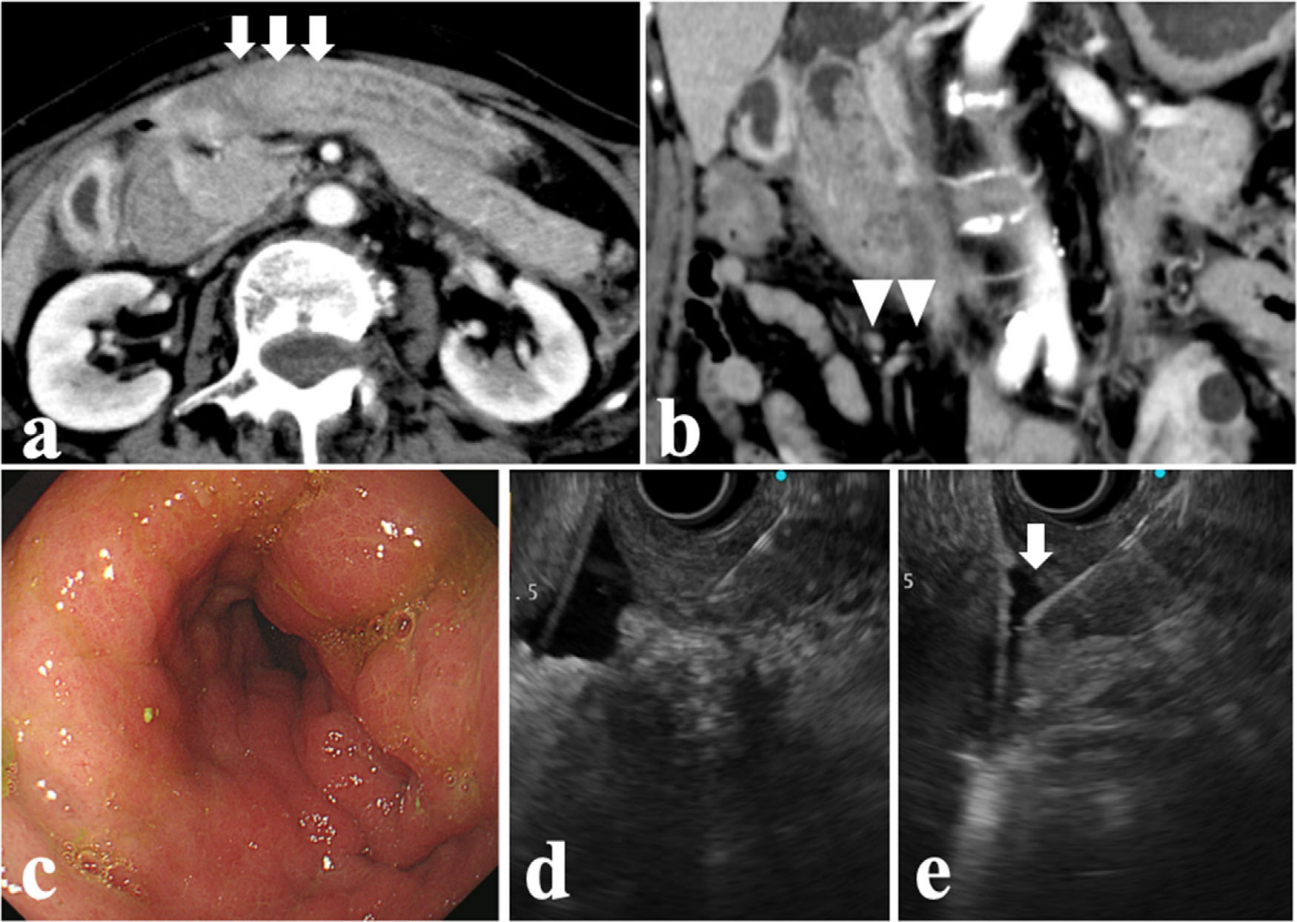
Findings of investigations performed in a 69‐year‐old female patient: (a) computed tomography (CT) findings showing a circumferential thickening of the gastric wall at the antrum (arrow); (b) multiple nodules were also observed in the abdominal cavity (arrowheads); (c) esophagogastroduodenoscopy (EGD) findings showing luminal rigid stricture without mucosal erosion and ulcers; (d) endoscopic ultrasound‐guided fine‐needle biopsy (EUS‐FNB) was performed to obtain a sample from the thickened gastric wall; (e) ascites were also aspirated (arrow)
